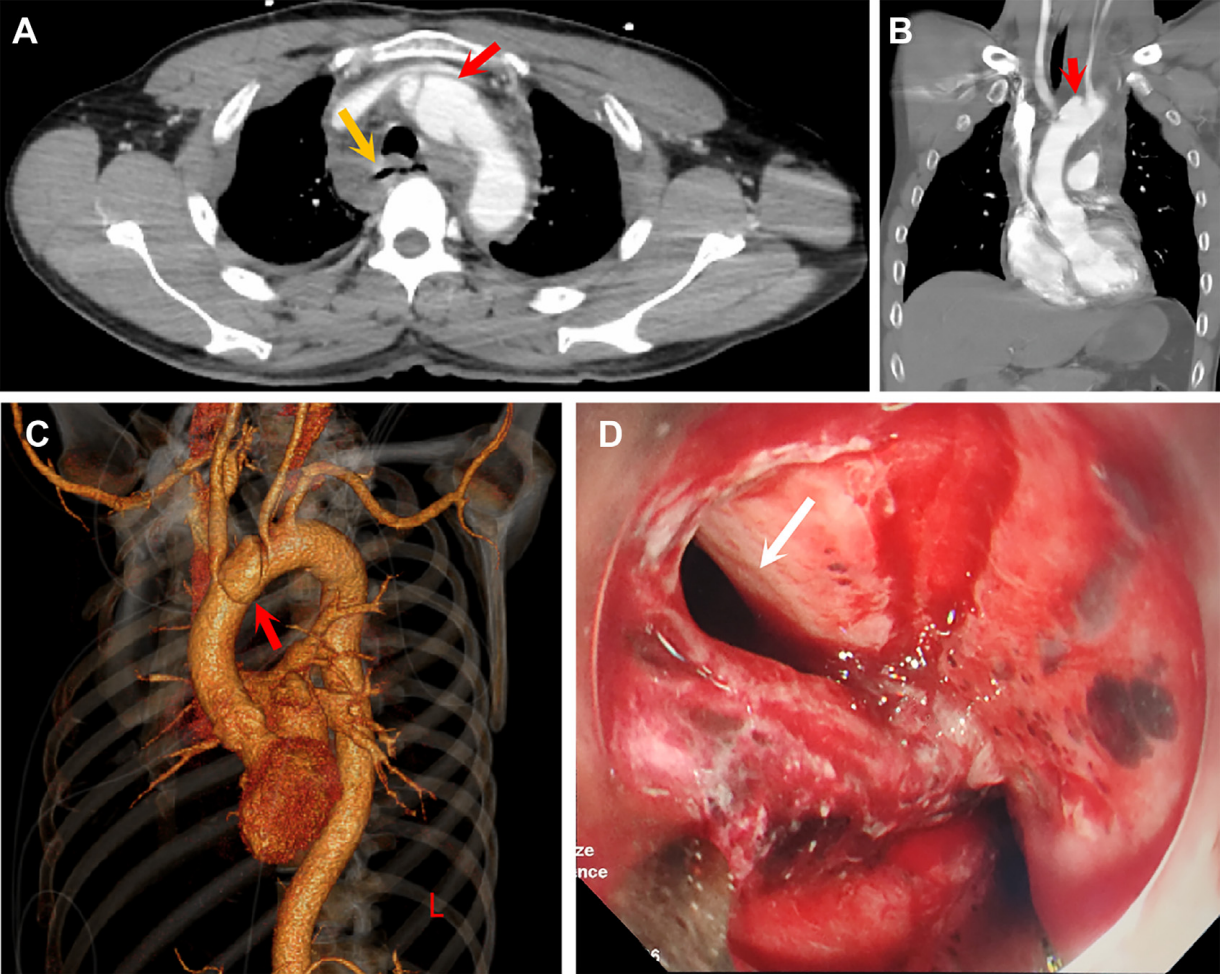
(A-C) Preoperative chest computed tomography showing laceration of the aortic arch (red arrow) and discontinuity of the esophageal lumen (yellow arrow). (D) Esophageal rupture on upper endoscopy (arrow).
